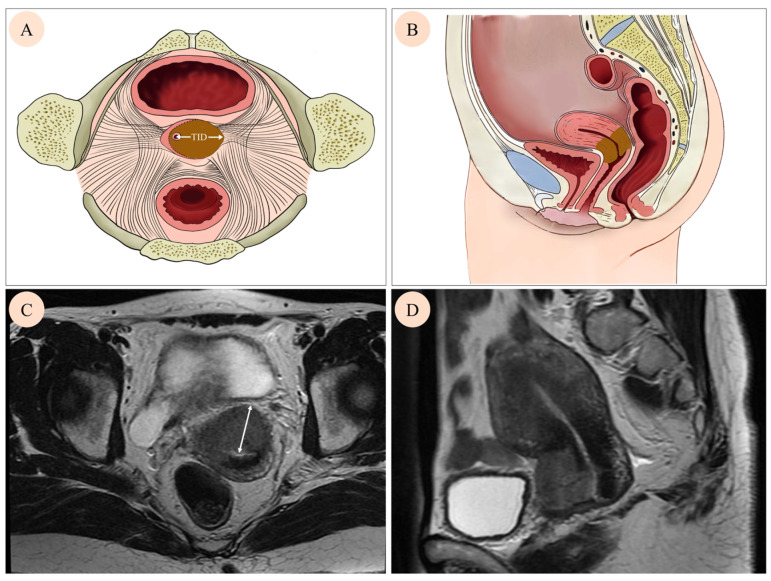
Cervical tumor growth on different images. A mass in the cervix was found by axial image (A), sagittal image (B), axial T2 weighted MRI image (C), and sagittal T2 weighted MRI (D). Tumor invasion distance (TID) was defined as the distance from the center of the cervical canal to the farthest edge (white arrow on (A,C)).
